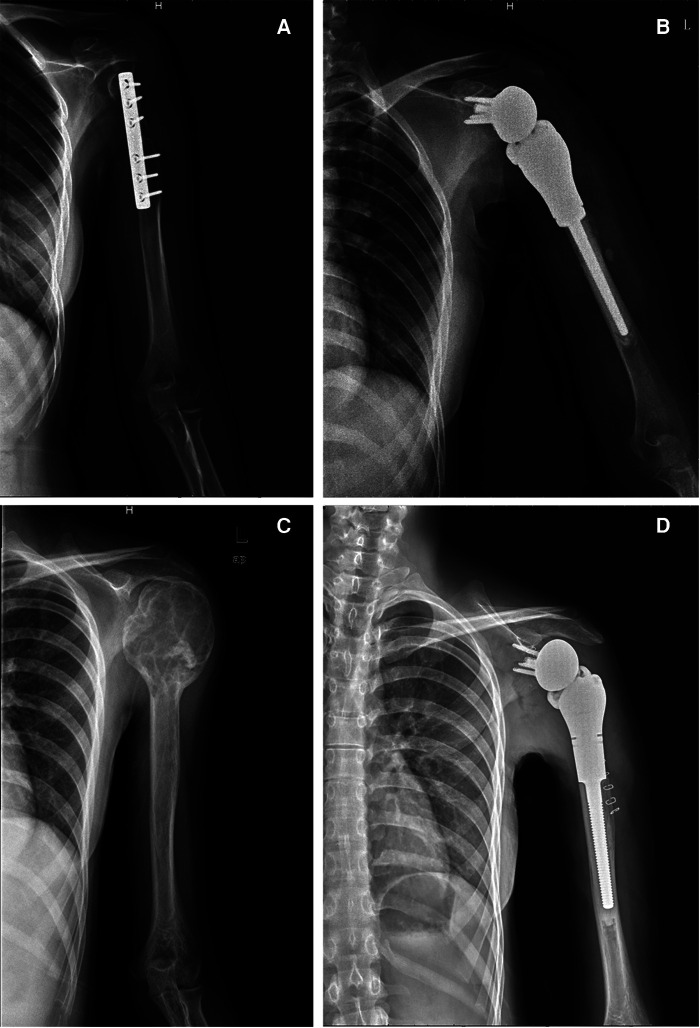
Imaging of two typical patients. (A) Preoperative x-ray of typical patient 1. (B) Postoperative x-ray of typical patient 1. (C) Preoperative x-ray of typical patient 2. (D) Postoperative x-ray of typical patient 2.
